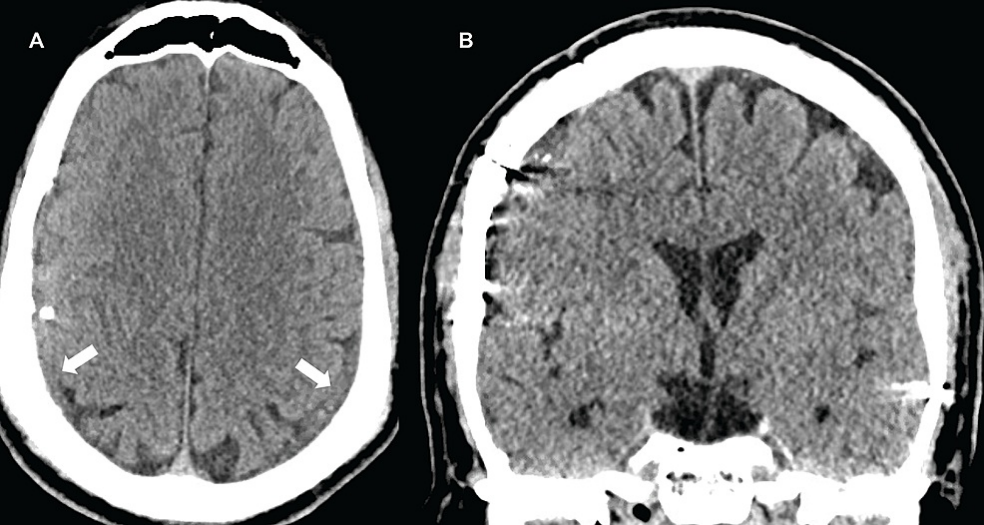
Six-week postoperative follow-up, non-contrast computed tomography of the head. (A) Axial plane. (B) Coronal plane. Minimal residual bilateral subdural hematomas (arrows) following surgical evacuation and middle meningeal artery embolization.
